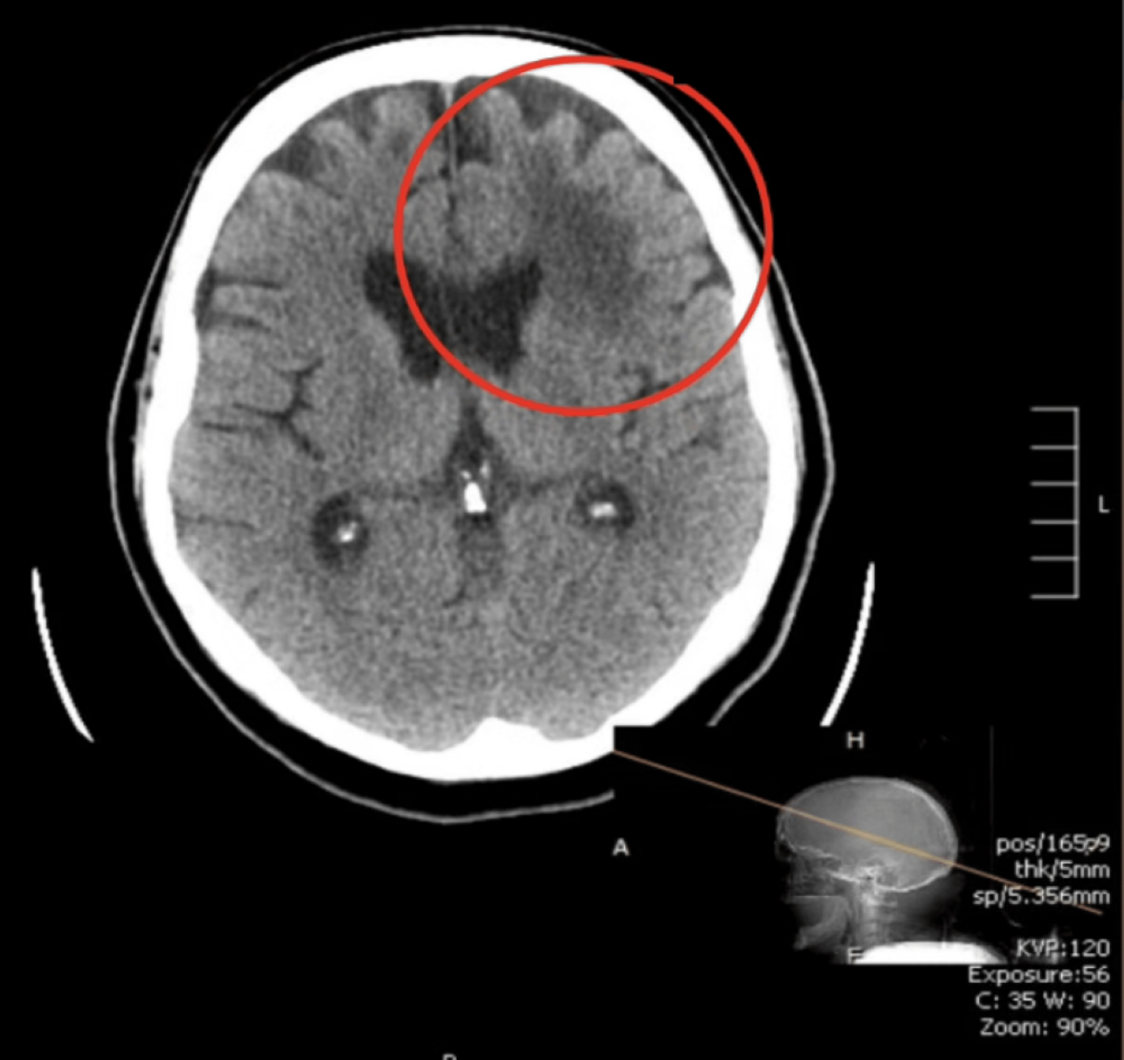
CT scan of the head showing an area of hypodensity in the left frontal lobe, marked with a circle.
